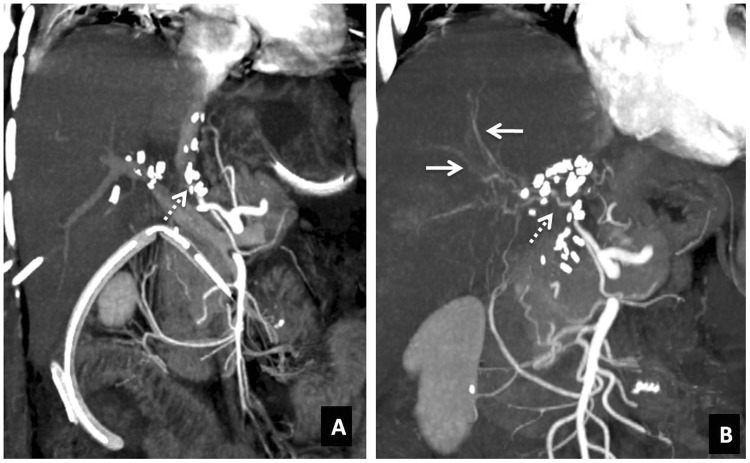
Hepatic artery thrombosis. (A) Coronal-oblique MIP contrast-enhanced CT images acquired in the arterial phase demonstrating abrupt termination of the hepatic artery (HA) at the anastomotic site (interrupted arrow) with non-visualization of the intrahepatic branches of HA. (B) Post-thrombolysis image showing opacification of the segment of the HA beyond the anastomosis (interrupted arrow) as well as the intrahepatic arteries (solid arrows).
